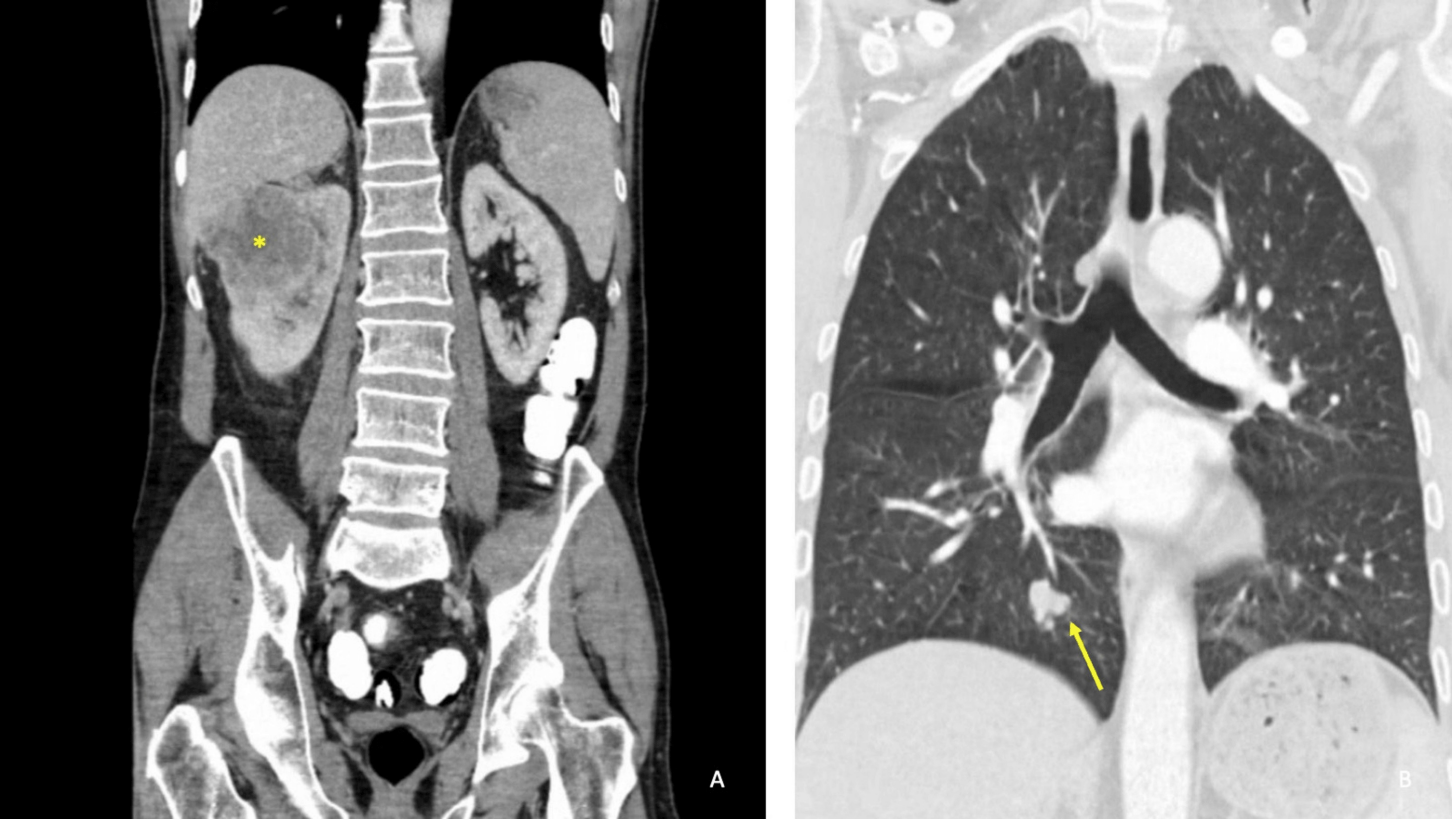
Computed tomography scan of the abdominopelvic (A) and chest (B) region. (A) Coronal CT image of the abdomen showing a renal tumor (*) located at the right upper pole, measuring 7 cm. (B) Coronal CT image of the chest showing lung metastasis (Yellow arrow).
